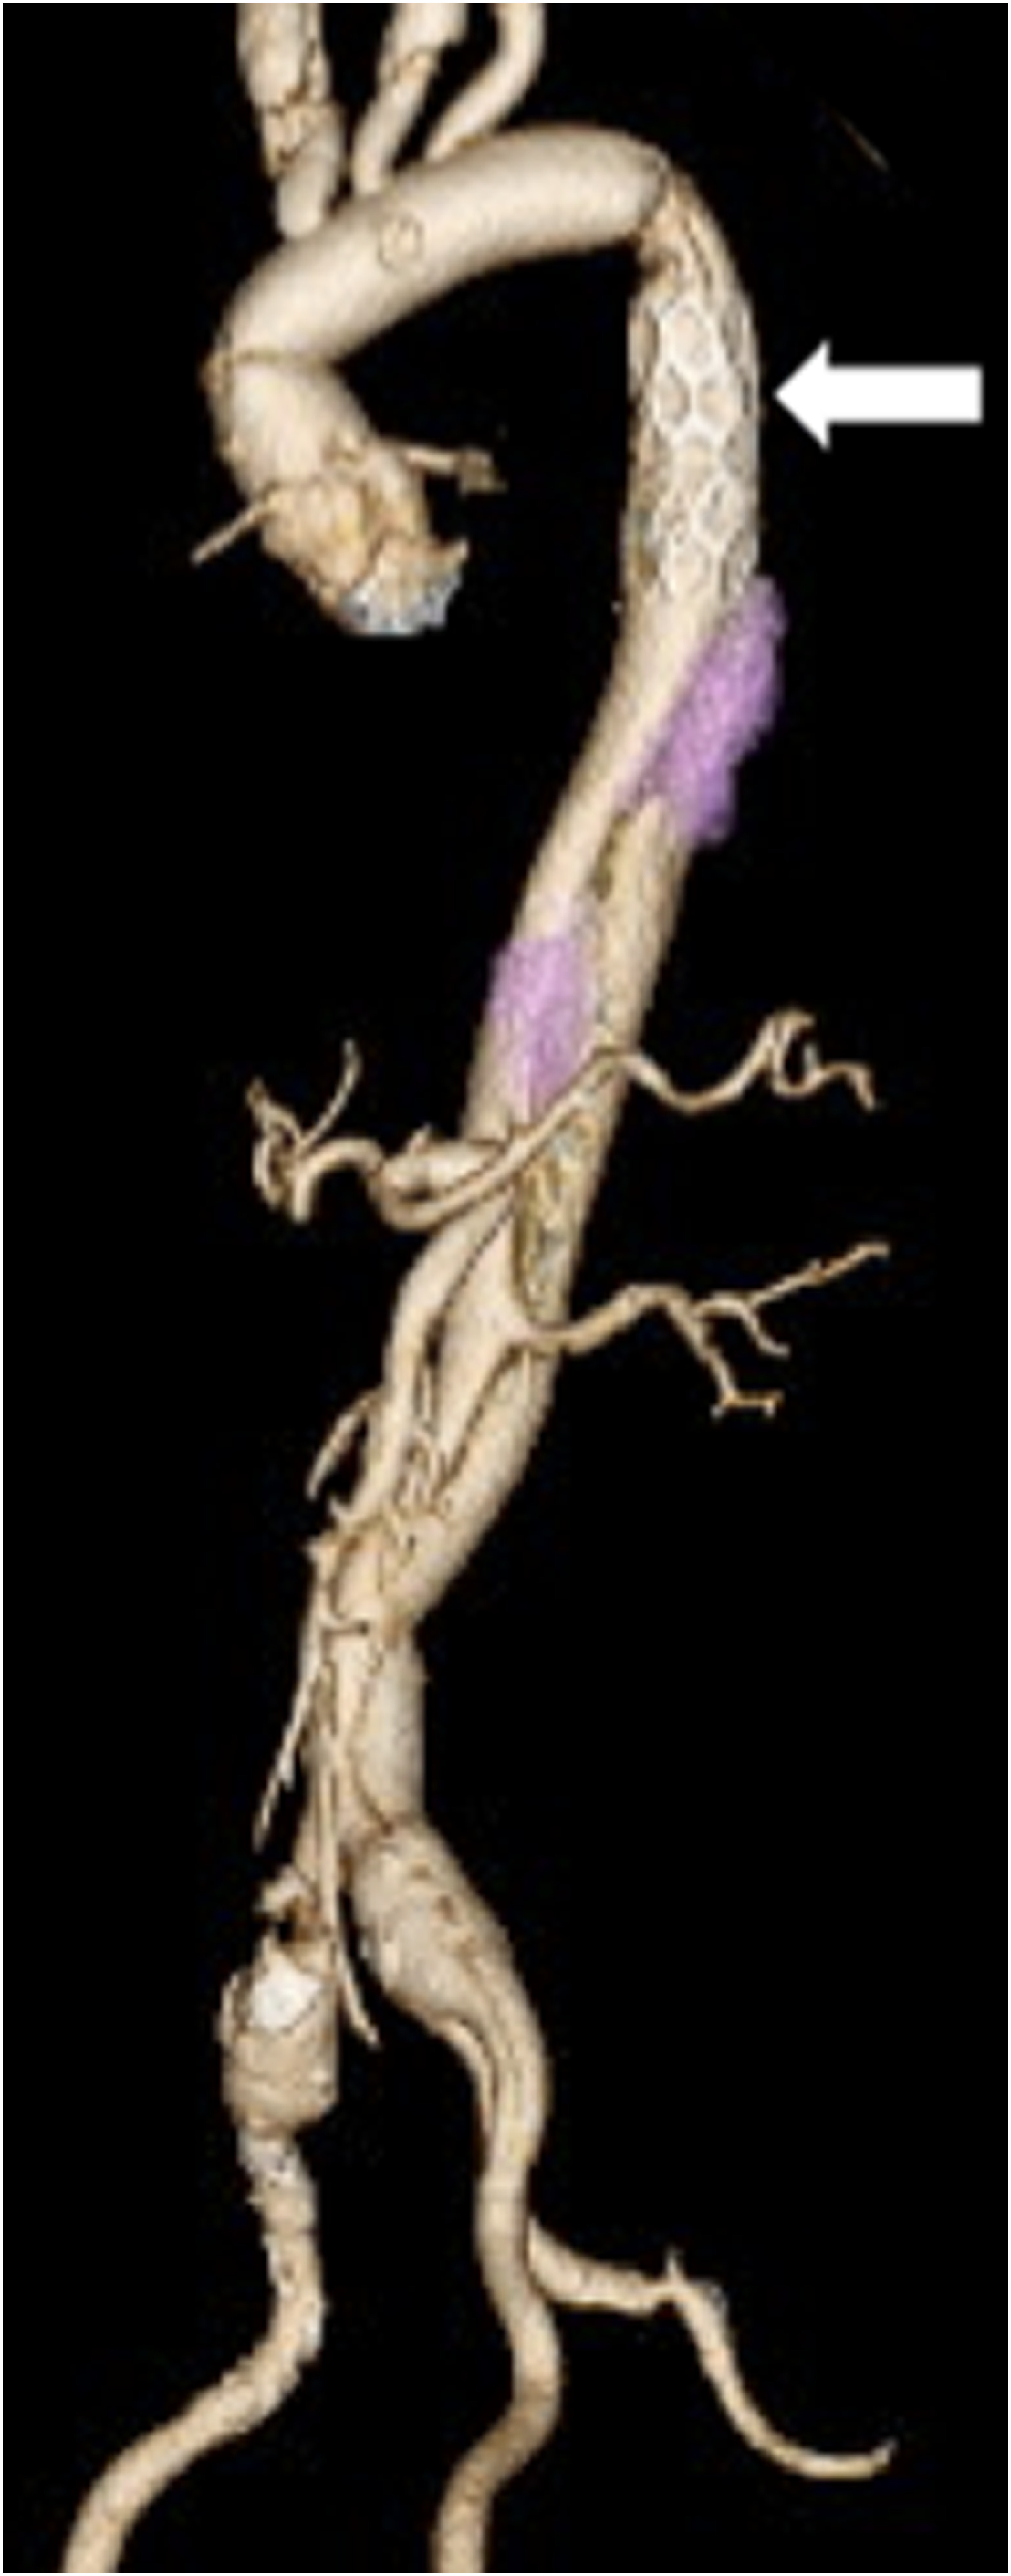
Fig. 3 Postoperative computed tomography scan shows valve-sparing root replacement and total arch replacement using frozen elephant trunk, with closure of the false lumen in the proximal descending aorta (arrow).
